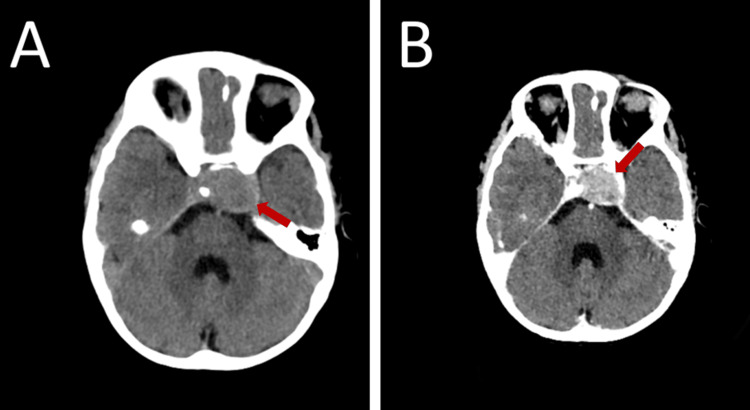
Pre-operative axial pre-contrast CT (A) shows a sellar hypodense lesion (red arrow) extending to the left cavernous sinus with bone changes. After IV contrast injection (B), the lesion (red arrow) shows enhancement. CT = computed tomography, IV = intravenous.
